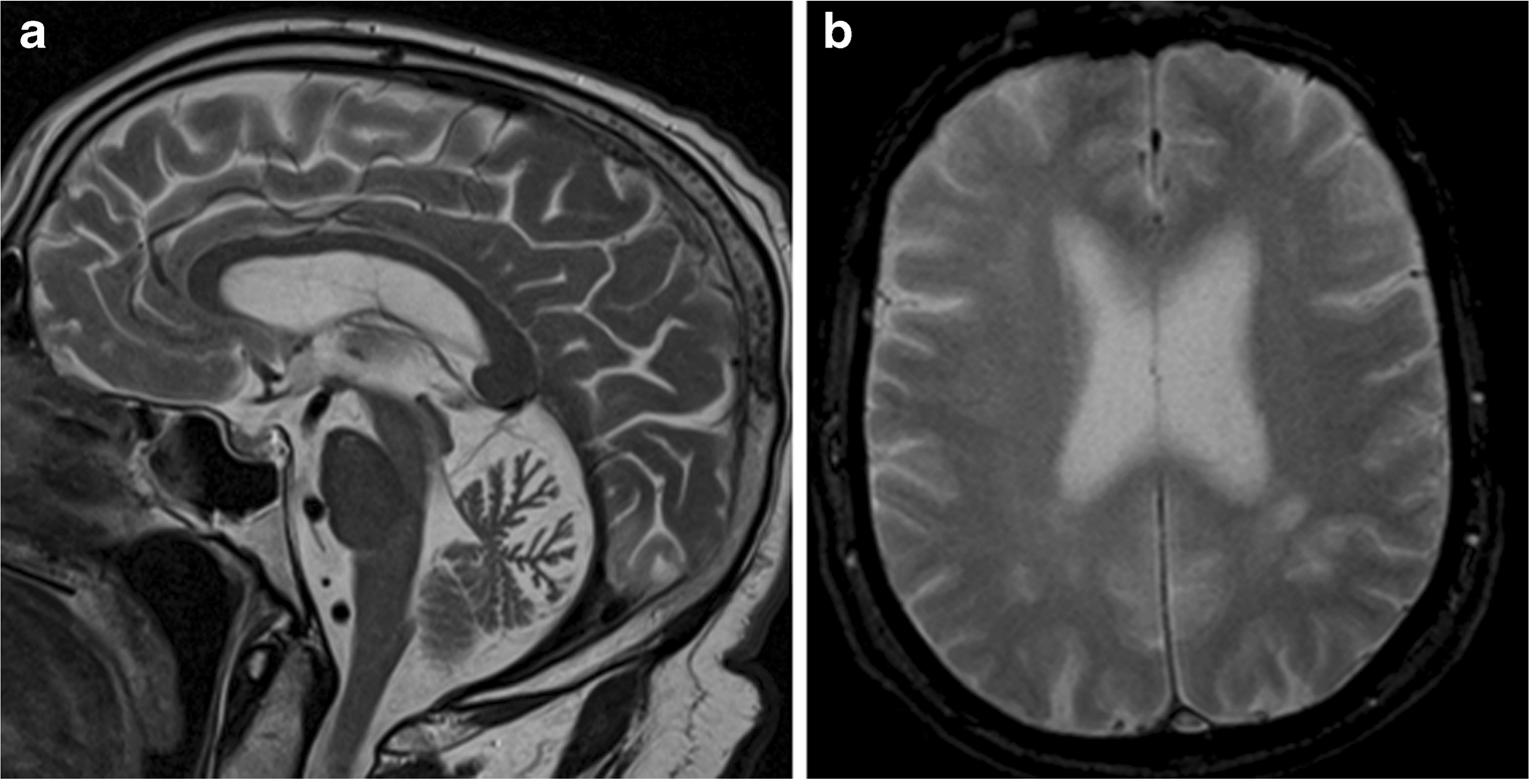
Brain MRI of patient III:1. a Midsagittal T2-weighted image displays moderate vermis atrophy. b Coronal T2-weighted image showing periventricular and deep white matter hyperintensities
